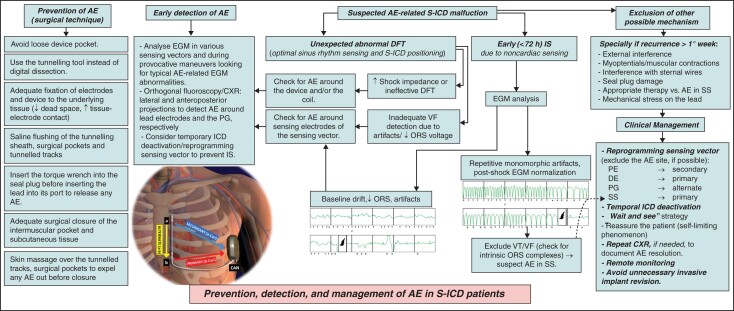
Prevention and management of S-ICD malfunctions possibly due to air entrapment. A proposed approach for prevention, detection, differential diagnosis, and management of AE and possible related malfunctions in S-ICD patients. AE, air entrapment; CXR, chest X-ray; DE, distal electrode; DFT, defibrillation threshold test; EGM, electrogram(s); IS, inappropriate shock(s); PE, proximal electrode; PG, pulse generator; S-ICD, subcutaneous ICD; SS, set screw; VF, ventricular fibrillation; VT, ventricular tachycardia.
